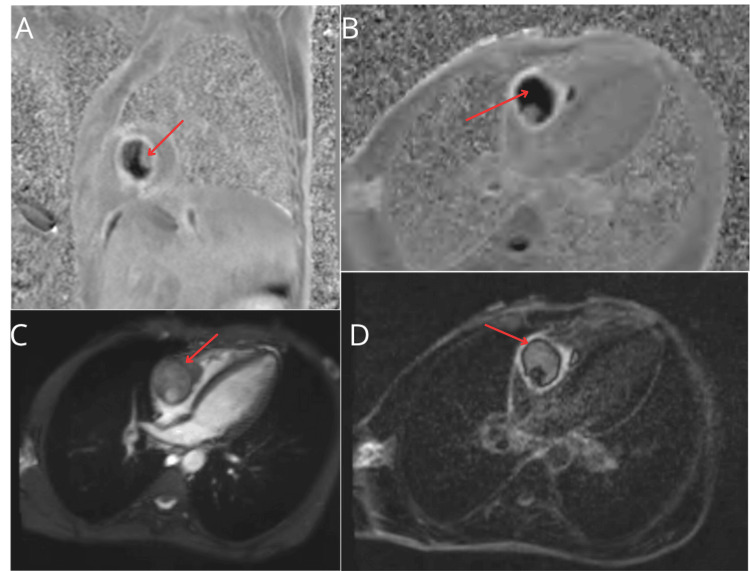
Cardiac MRI: (A-D) Right atrial mass with features of malignant thrombus continuous with IVC thrombus (arrows). Images were obtained from the authors’ institutional database. IVC, inferior vena cava
